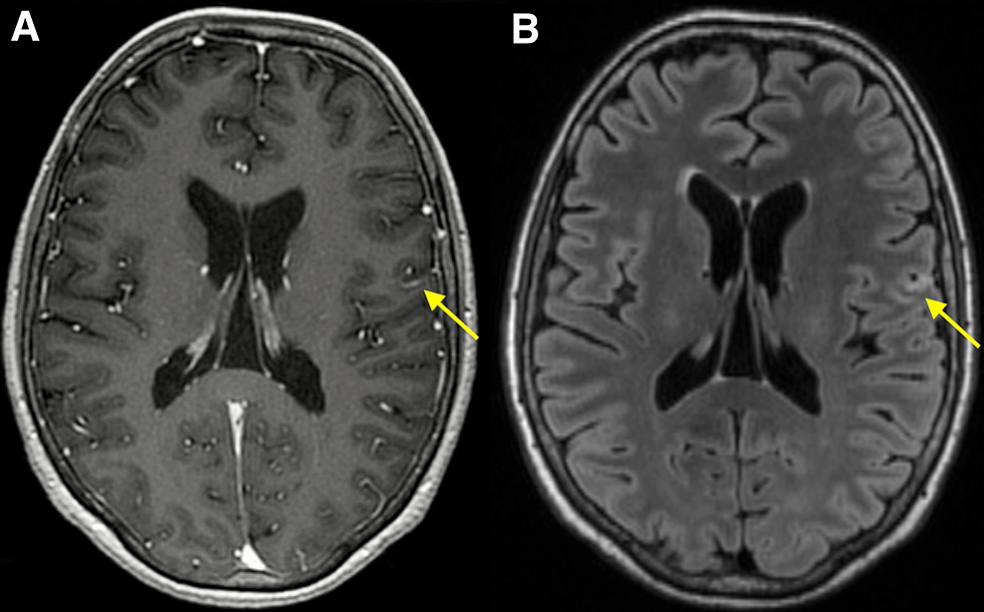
MRI 3 months after Gamma Knife stereotactic radiosurgery. Axial contrast-enhanced T1-weighted (A) and T2-weighted FLAIR brain MRI (B) obtained 3 months after Gamma Knife stereotactic radiosurgery demonstrating interval significant decrease in size of the enhancing left frontal lesion with a significant decrease in surrounding vasogenic edema. FLAIR: Fluid-attenuated inversion recovery
